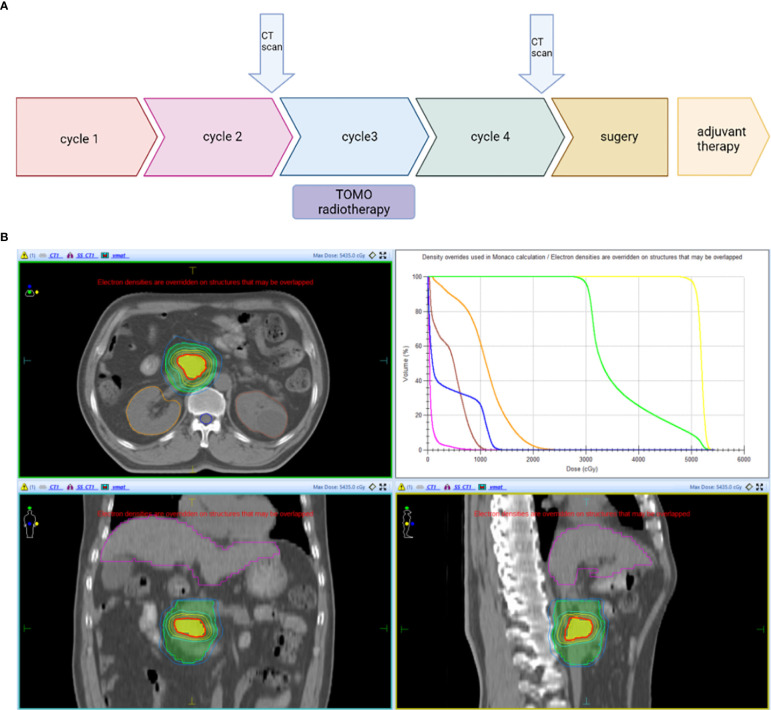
(A) Timeline of the treatment: Every 3 weeks one cycle: Tislelizumab (200 mg, on day 1), gemcitabine (1,000 mg/m^2^, on days 1 and 8), and nab-paclitaxel (125 mg/m^2^, on days 1 and 8). (B) Radiotherapy: PTV: 3 Gy * 10f; PGTV: 5 Gy * 10f. CT scan, Computed Tomography scan; PTV, planning target volume; PGTV, planning gross tumor volume.
